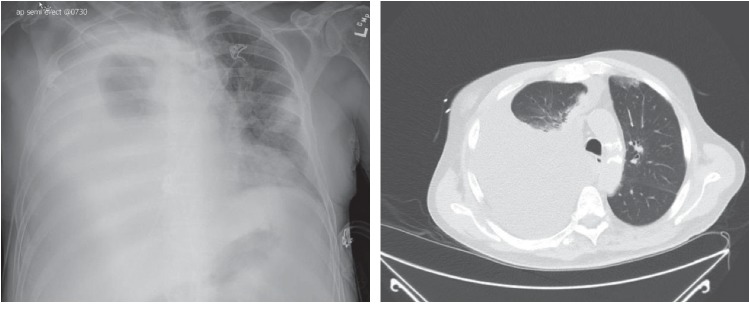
Chest X-ray and chest CT scan showing a right-sided pleural effusion.
